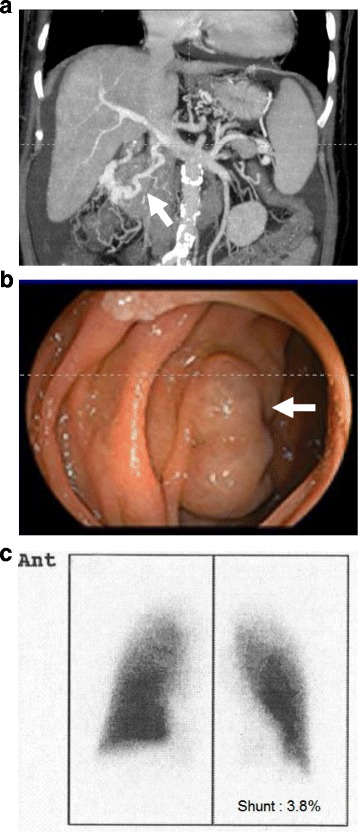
A preoperative coronal section image of CT scan and right spleno-renal shunt (a and white arrow, respectively). A preoperative image of upper gastrointestinal endoscopy and varix (b and white arrow, respectively). A preoperative radiolabeled technetium macroaggregated-albumin (99mTc-MAA) perfusion scan (c anterior image) showing the absence of right to left shunt (3.8%, similar to background)
